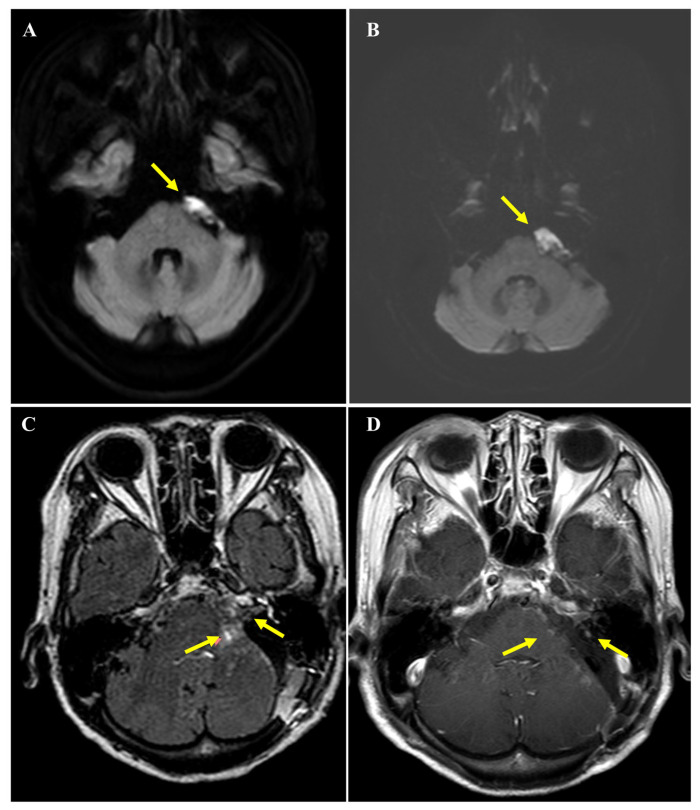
Serial magnetic resonance imaging (MRI) findings before surgery and prior to Gamma Knife radiosurgery. (A) Axial diffusion-weighted MRI demonstrating a well-defined lesion at the left cerebellopontine angle (arrow) with imaging features consistent with an intracranial epidermoid cyst, measuring approximately 2.3 × 1.1 cm, without significant mass effect on adjacent brainstem structures. (B) Follow-up axial diffusion-weighted MRI showing equivocal interval change with subtle enlargement of the left cerebellopontine angle lesion (arrow) to approximately 2.6 × 1.1 cm, while no definite mass effect or hydrocephalus is observed. (C) Axial post-contrast T2 fluid-attenuated inversion recovery MRI obtained on postoperative brain MRI before Gamma Knife radiosurgery demonstrating residual enhancing tumor components at the left cerebellopontine angle and extension into the internal auditory canal (arrows) following subtotal resection. Mild residual mass effect on the left pons and slight displacement of the ipsilateral facial and vestibulocochlear nerve complex are also noted. (D) Axial contrast-enhanced T1-weighted spin-echo MRI obtained 18 months after Gamma Knife radiosurgery demonstrating marked reduction of the residual tumor at the left cerebellopontine angle and decreased extent of enhancing lesions within the left internal auditory canal (arrows), consistent with radiographic response and no evidence of disease progression.
